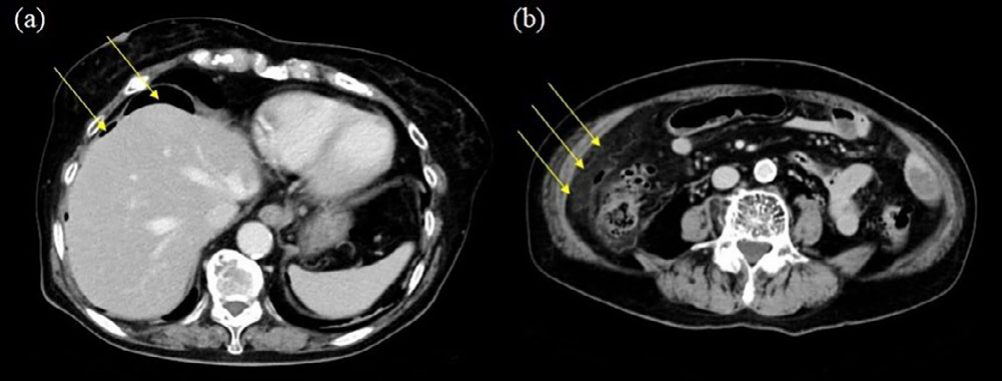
Computed tomography reveals free air on the liver surface (a: arrows) and peri‐cecal inflammation with small air bubbles (b: arrows)
